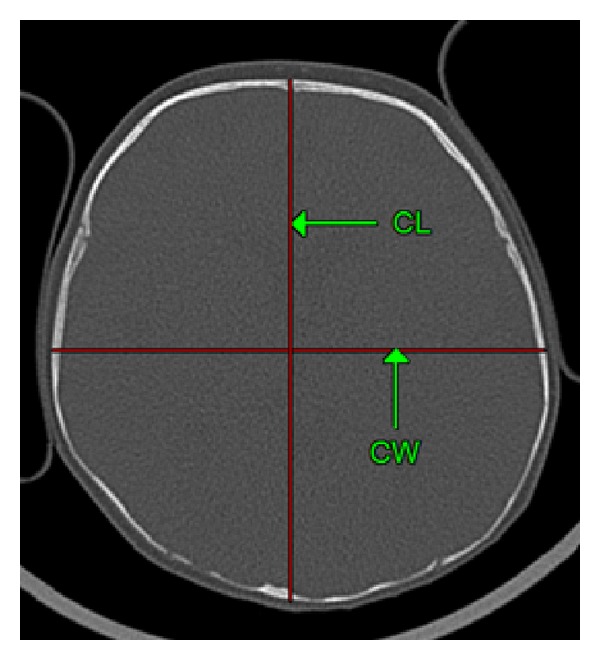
Measurements of the cranium (axial CT scan; child age: 5 months): CL: cephalic length, CW: cephalic width. Cephalic index = CW/CL × 100.
